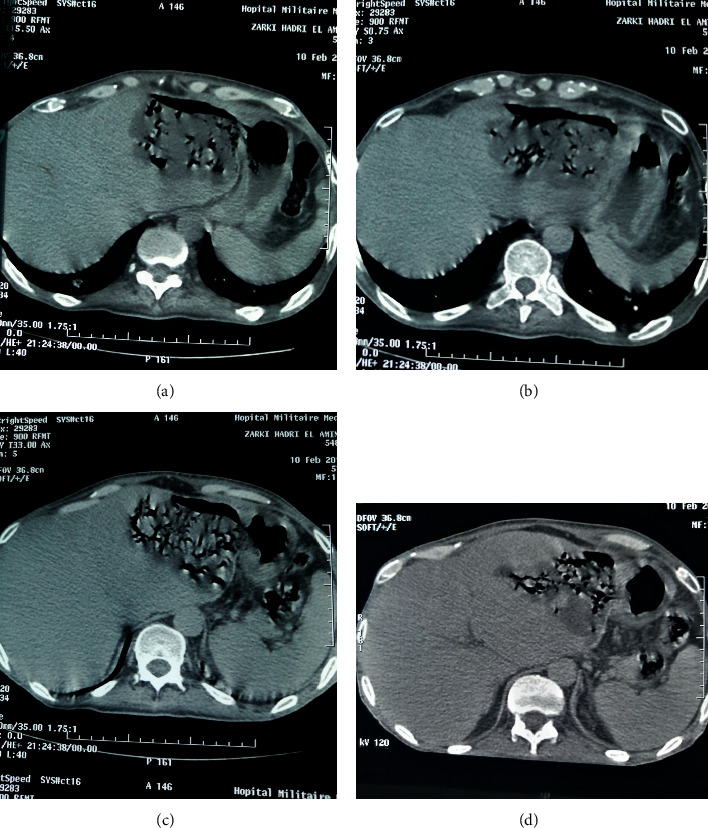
Preoperative CT scan showing (a, b) liver cystic mass containing multiple air foci; (c, d) hepatogastric fistula.
